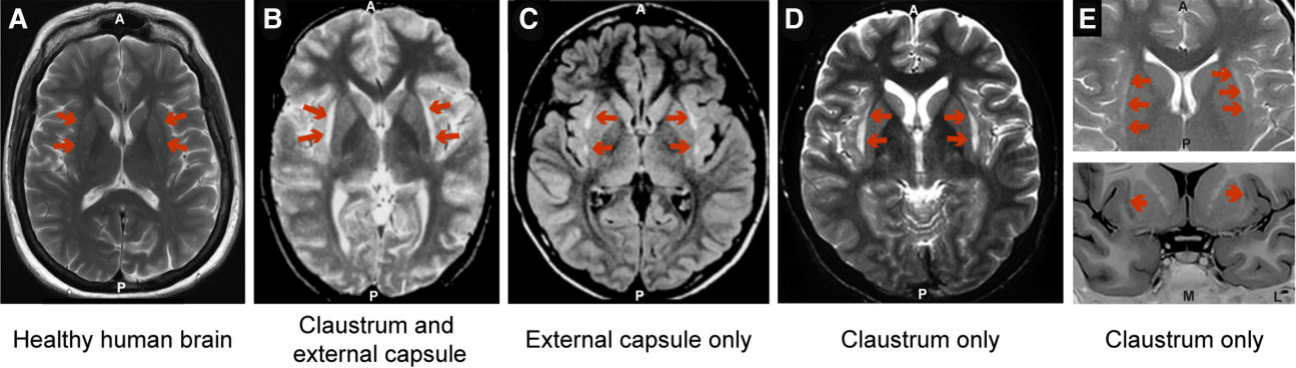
Similar lesions have been described as affecting either the claustrum, the external capsule, or both. (A) Representative T_2_-weighted image of a healthy human brain. (B) T_2_-weighted image showing a lesion reported as affecting the claustrum and external capsule. Reprinted from Sperner *et al*.^45^ with permission from Springer Nature ©1996. (C) Fluid-attenuated inversion recovery (FLAIR) image showing a lesion reported as affecting the external capsule. Reprinted from Mumoli *et al*.^43^ with permission from Springer Nature ©2014. (D) T_2_-weighted image showing a lesion reported as affecting the claustrum. Reprinted from Ishii *et al*.^42^ with permission from American Society of Neuroradiology ©2011. (E) T_2_-weighted image (*top*) and T_1_-weighted image (*bottom*) showing a lesion reported as affecting the claustrum. Reprinted from Silva *et al*.^44^ with permission from Springer Nature ©2018.
